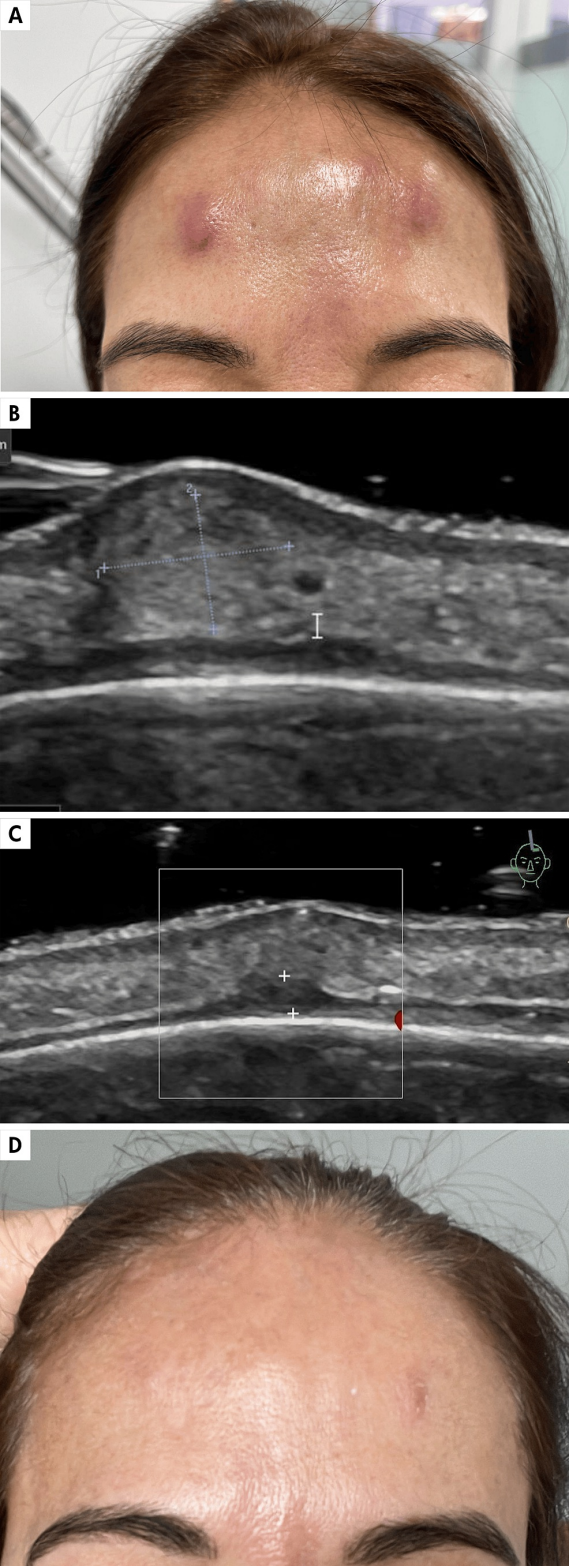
Inflammatory nodules seen with high-resolution gray-scale ultrasound. A. Inflammatory nodules in the frontal region after botulinum toxin injection. B. High-resolution gray-scale ultrasound image, axial view, showing an inflammatory nodule in the dermal-subcutaneous layer, which is hyperechoic with partially defined borders (delimited by calipers +), causing displacement of the epidermis superiorly. C. High-resolution gray-scale ultrasound image, axial view, immediately below the inflammatory nodule (n), there is a thickening of the frontalis muscle with irregular borders suggestive of myositis (delimited by calipers +). D. Clinical image of the frontal region after completing treatment showing near-complete resolution of the nodules.
